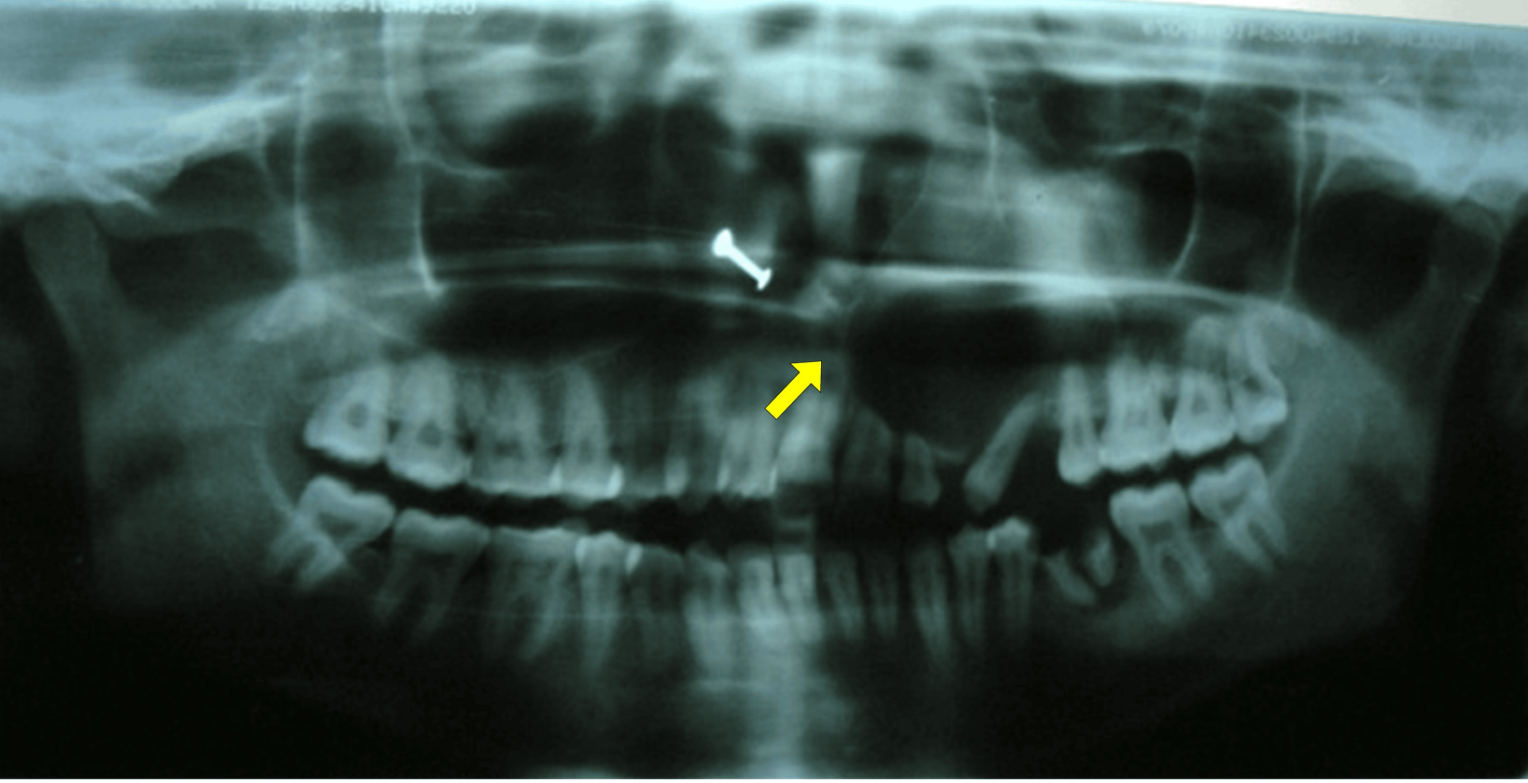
Orthopantomogram showing solitary well-defined radiolucency with a cortical margin (lesion indicated by the arrow)
